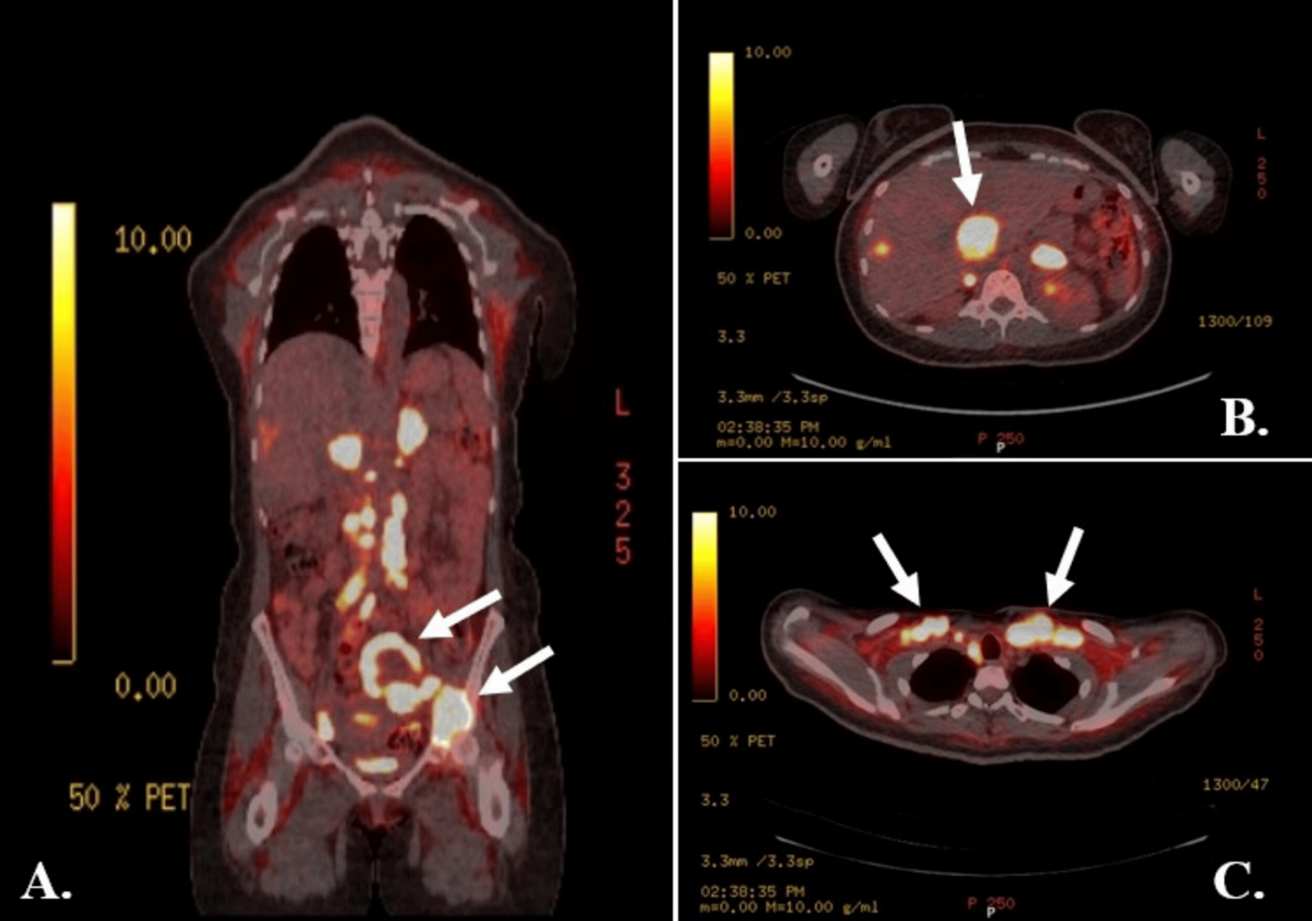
PET-CT images demonstrating disseminated metastatic disease. (A) Coronal section showing a large hypermetabolic left pelvic mass with extensive FDG-avid lymphadenopathy above and below the diaphragm, and involvement of the left acetabulum (arrows). (B) Axial section showing an FDG-avid metastatic deposit in the right hepatic lobe (arrow). (C) Axial section demonstrating bilateral mediastinal lymphadenopathy (arrows). PET-CT: positron emission tomography-computed tomography; FDG: fluorodeoxyglucose
